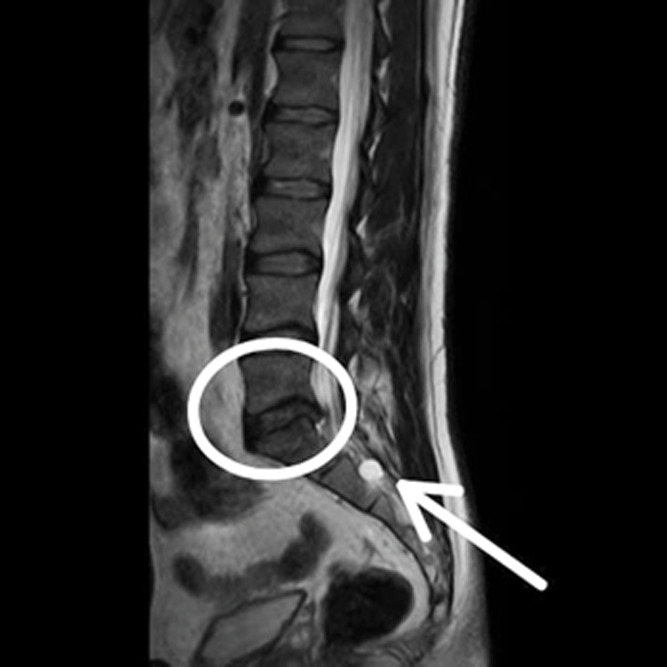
sagittal T2-weighted magnetic resonance imaging demonstrating a prominent sacral Tarlov cyst at the S1-S2 level with concurrent L5-S1 disc space narrowing and posterior disc bulge, T2 hyperintensity noted in the cerebrospinal fluid-filled perineural cyst, degenerative changes with disc height loss at the L5-S1 level, S/O double crush phenomenon affecting the S1 nerve root territory
